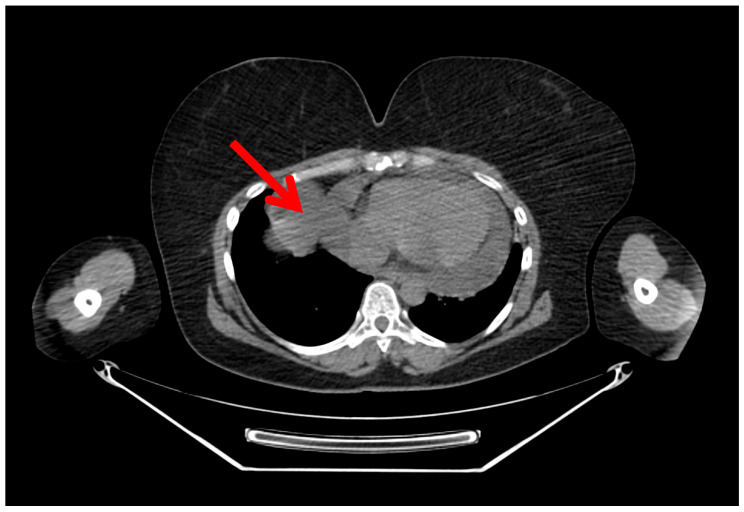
Axial view of the chest CT confirming fluid-filled sac-like connection to right pleural space (red arrow).
